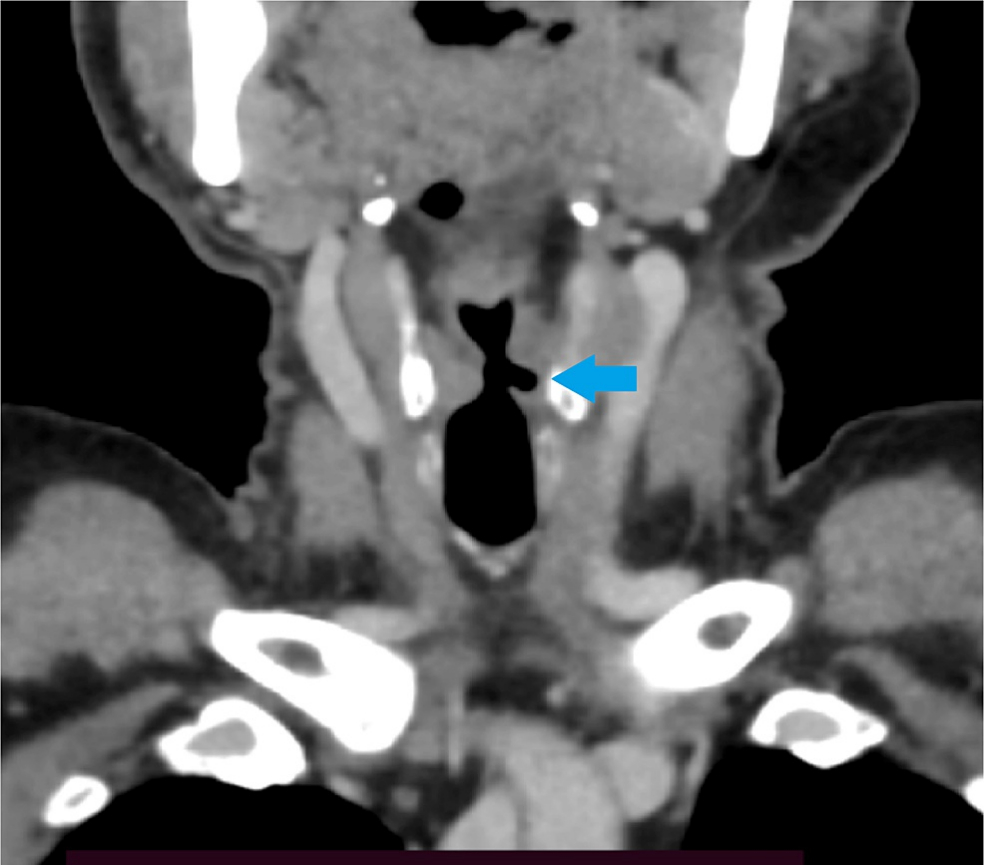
Contrast-enhanced computed tomography of the neck in the coronal section showing the dilated laryngeal ventricle, dilation of pyriform sinus, and lateralized true vocal fold on the left side consistent with the sail sign. Dilated left laryngeal ventricle (arrow).
